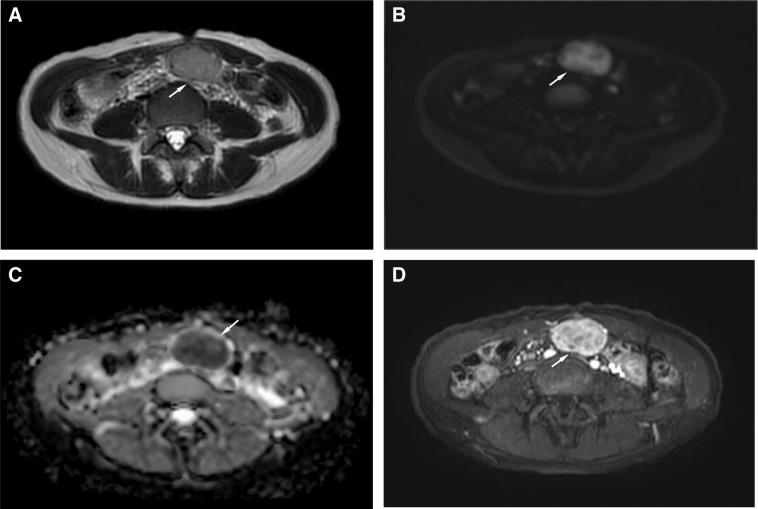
MRI shows T2 hyperintensity, diffusion restriction, and early enhancement. (A) T2-weighted imaging shows a well-defined, hyperintense mass (arrow). (B) Diffusion-weighted imaging demonstrates high signal intensity within the lesion (arrow). (C) The corresponding apparent diffusion coefficient (ADC) map shows low signal intensity, consistent with diffusion restriction (arrow). (D) Dynamic contrast-enhanced imaging reveals early enhancement of the tumor, suggestive of a hypervascular soft-tissue neoplasm (arrow).
